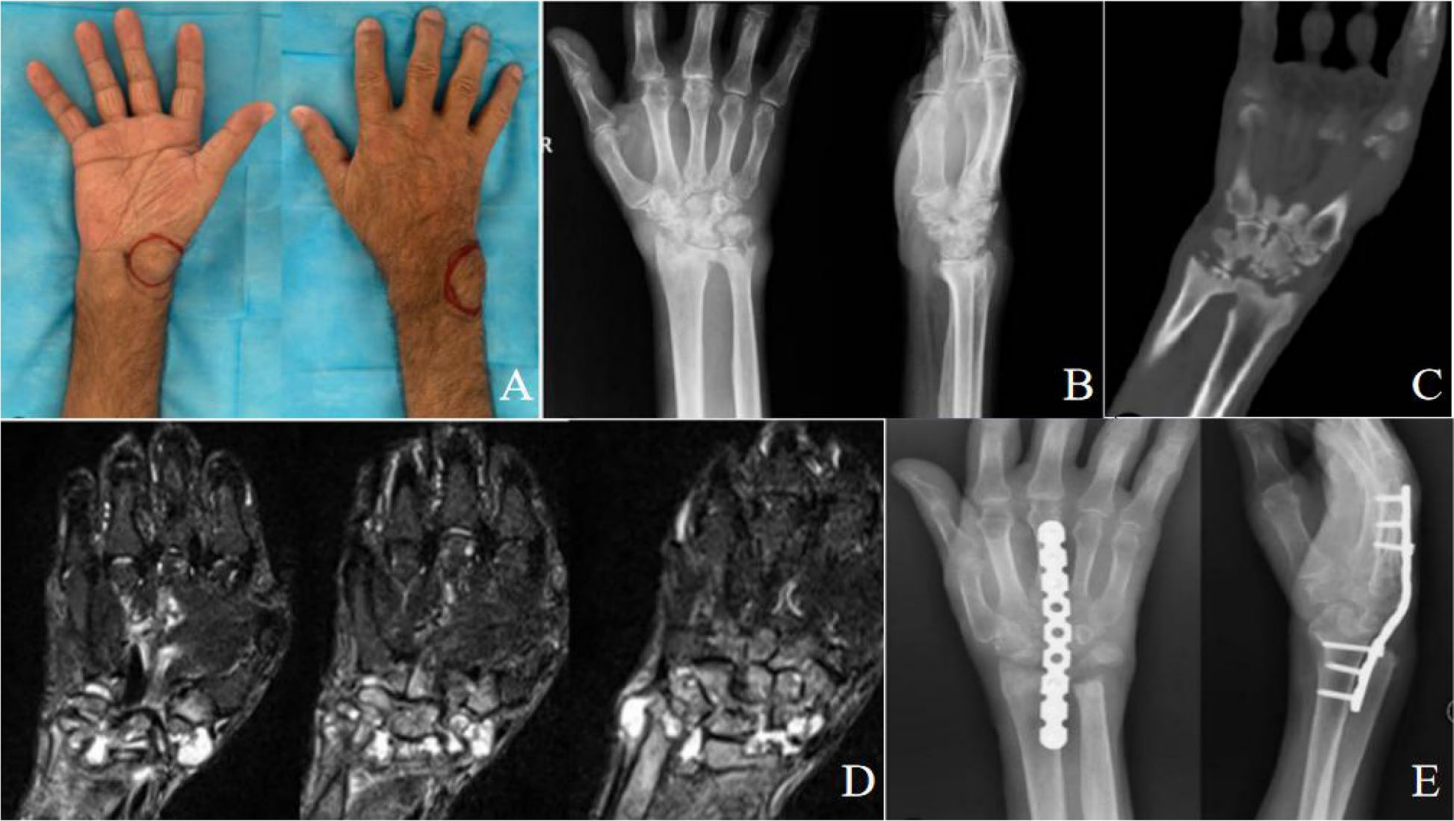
A 46-year-old male patient with wrist tuberculous. A. Swelling can be observed on the palmar and dorsal side of the right wrist. B,C. Anteroposterior, lateral X-ray, and CT scan of the right wrist showed an osteolytic lesion of the distal radius, distal ulna, and carpal bones. D, MRI images of the right hand showed significant osteolytic lesions in the distal ulna and carpal bones, and extensive necrotic degeneration of the synovium of the wrist joint. E, Anteroposterior and lateral X-rays showed 28 months after wrist joint fusion by plate fixation
